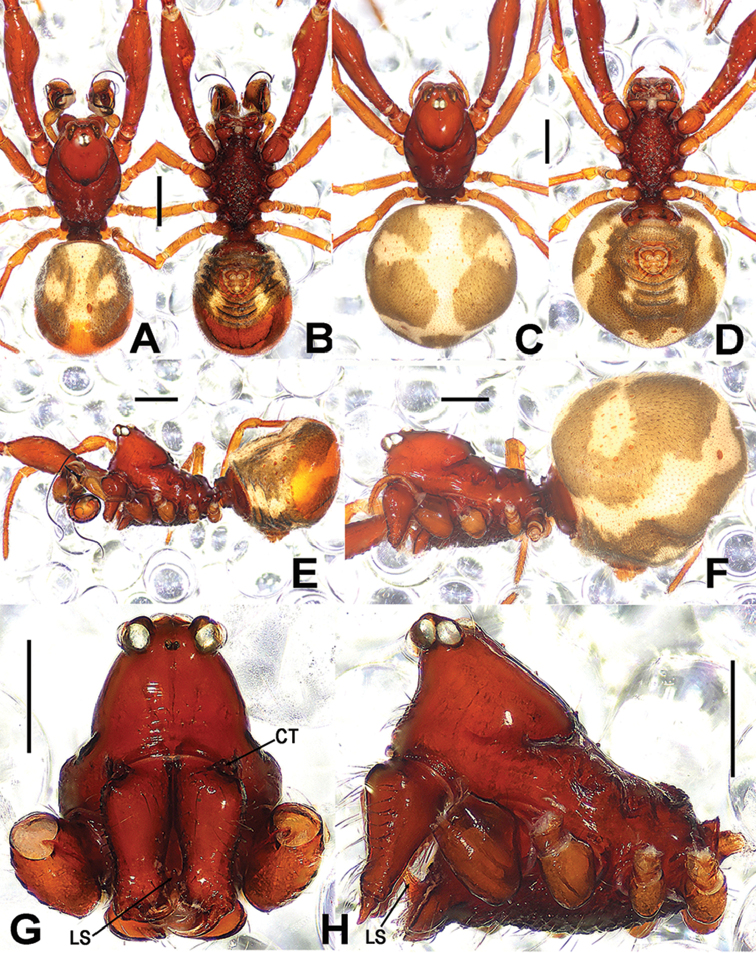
Sinanapislongituba* Lin & Li, 2012, A, B, E Male habitus C, D, F Female habitus G, H Prosoma (appendages omitted) A, C dorsal B, D ventral E, F, H lateral G anterior. Abbreviations: CT cheliceral tubercle; LS labial spur. Scale bars: 0.50 (A–F); 0.20 (G, H).
